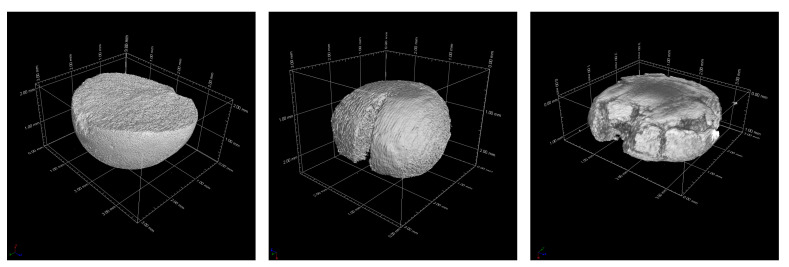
CT-rendered images of beads after compression test. Al_2_O_3__100-L (left), Nb_95-L (center), and Nb_100-L (right).
